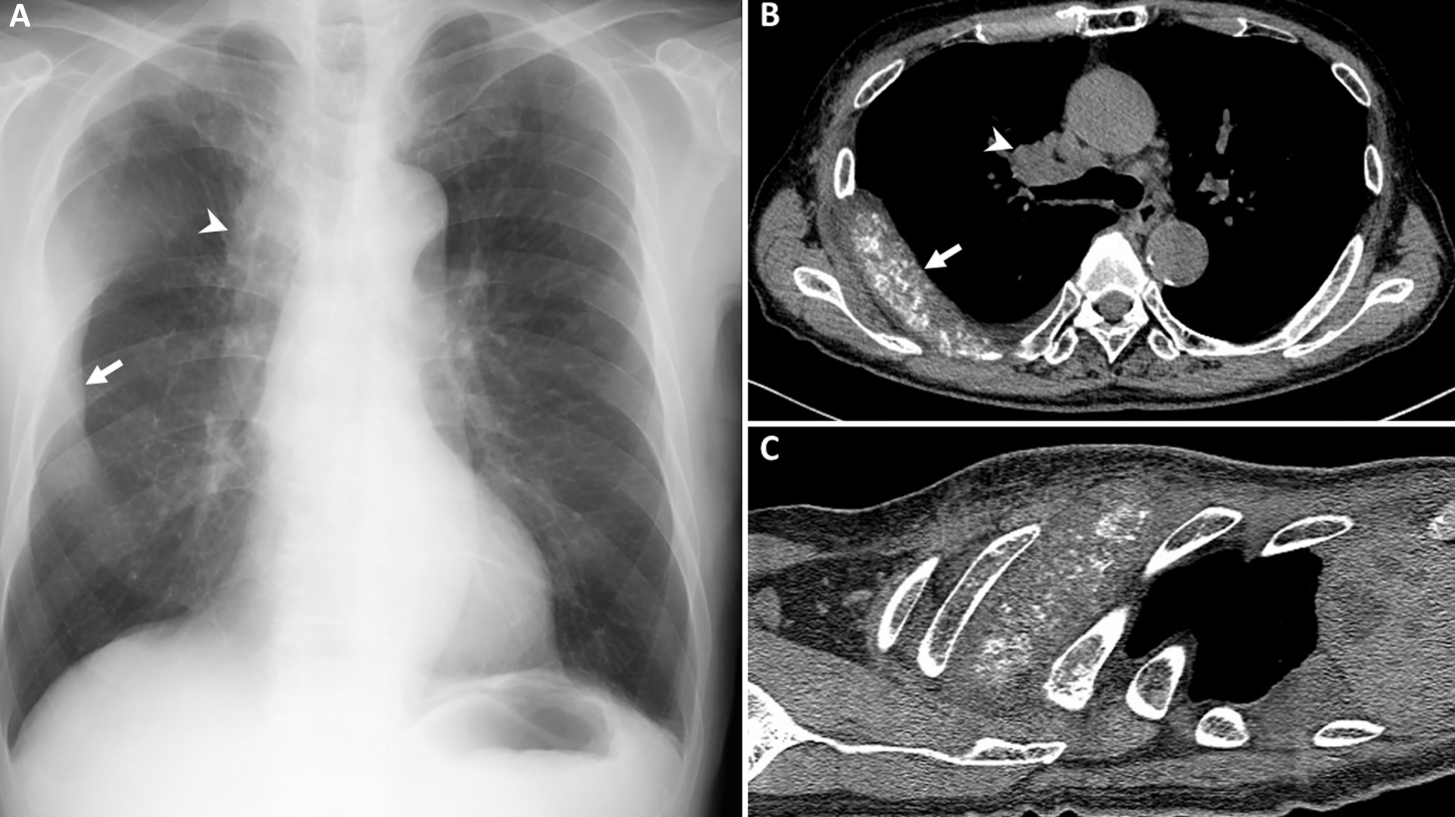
(A) Chest radiography showing a banana‐shaped opacity (arrow) and a tumorous shadow (arrowhead) in the right lung field. Axial (B) and sagittal (C) computed tomographic scans of the chest showing a mass in the upper lung lobe (arrowhead) and a fragmented and enlarged rib (arrow) due to metastasis.
